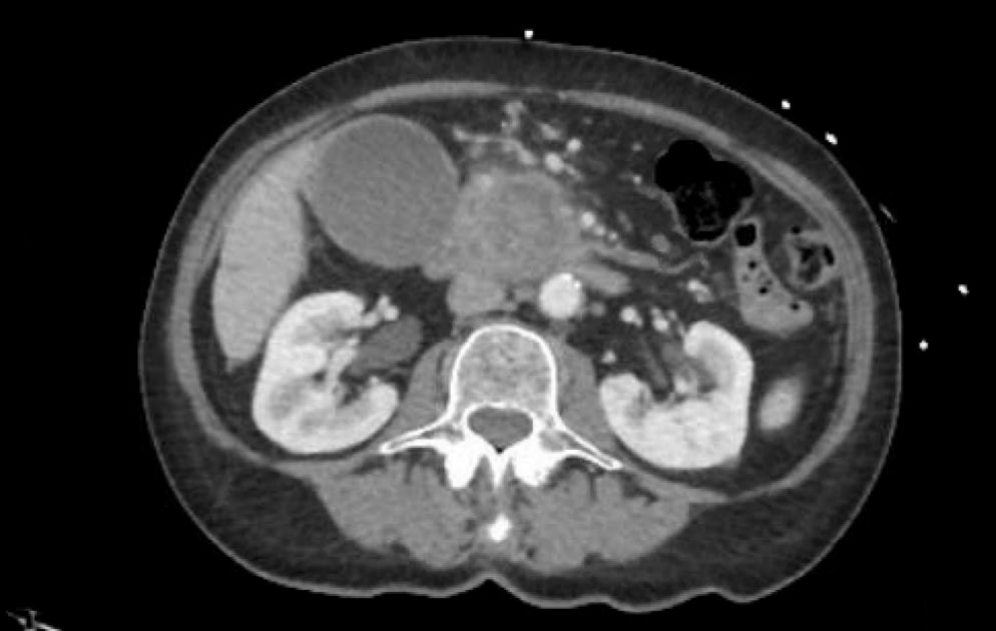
CT Abdomen showing pancreatic head mass with pancreatic ductal dilatation, consistent with pancreatic adenocarcinoma.
